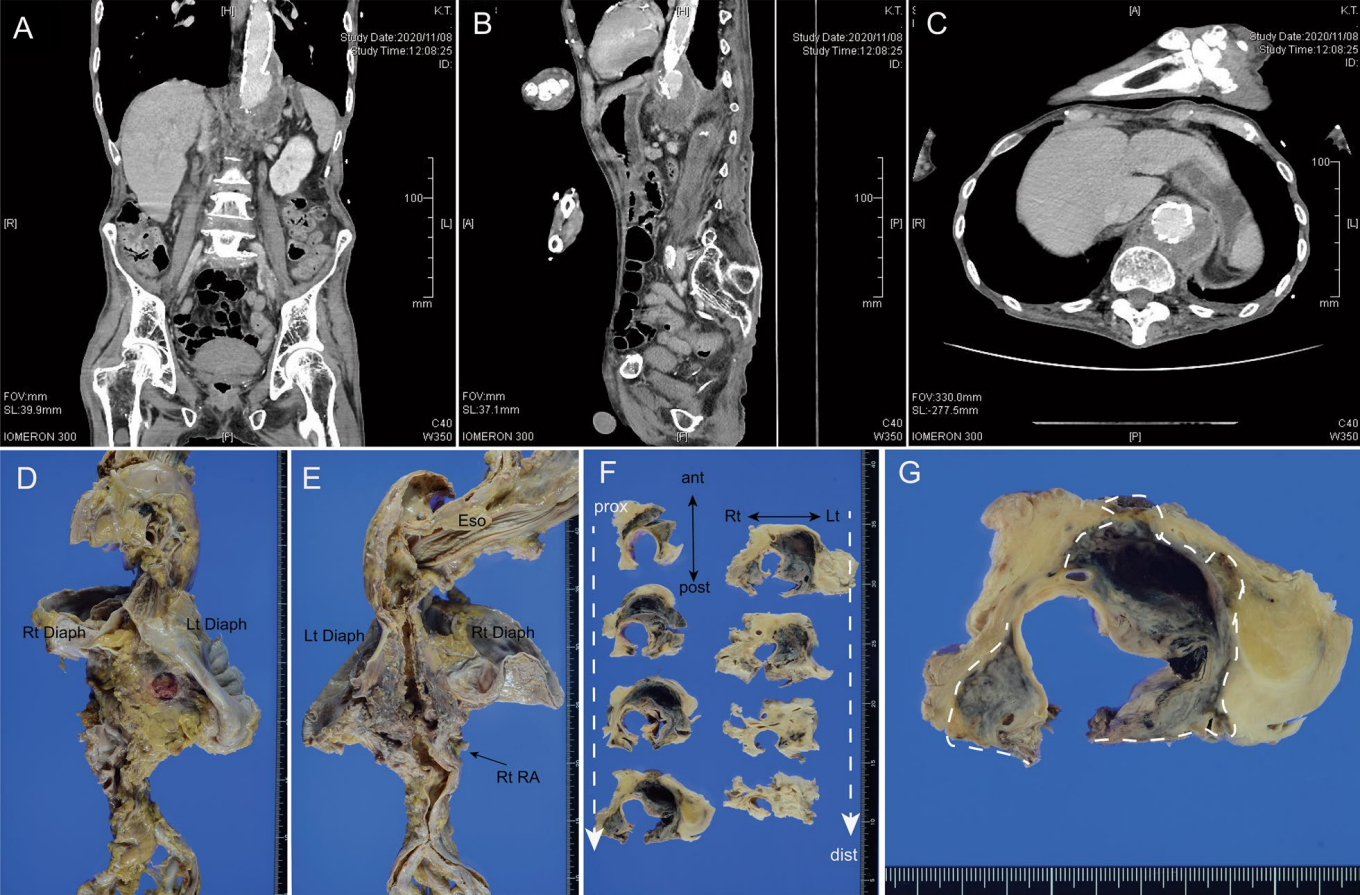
Radiological and macroscopic appearance of the patient in this case report. A–C Computed tomography scan of the abdomen and pelvis at admission. A Coronal section, B sagittal section, C axial section. D–G Macroscopic appearance of the infective aortic aneurysm. D, E Anterior (D) and posterior (E) views of the aorta and circumferential structure. Dilatation of the suprarenal aorta can be seen. *Rt Diaph* right diaphragm, *Lt diaphragm* left diaphragm, *Eso* esophagus, *Rt RA* right renal artery. F, G Transverse section of the suprarenal aorta. Partial loss of the aortic structure and saccular outpouching of the aneurysmal wall. Dotted line shows the outline of the aneurysmal wall. *Prox* proximal, *Dist* distal, *Ant* anterior, *Post* posterior, *Rt* right, *Lt* left
